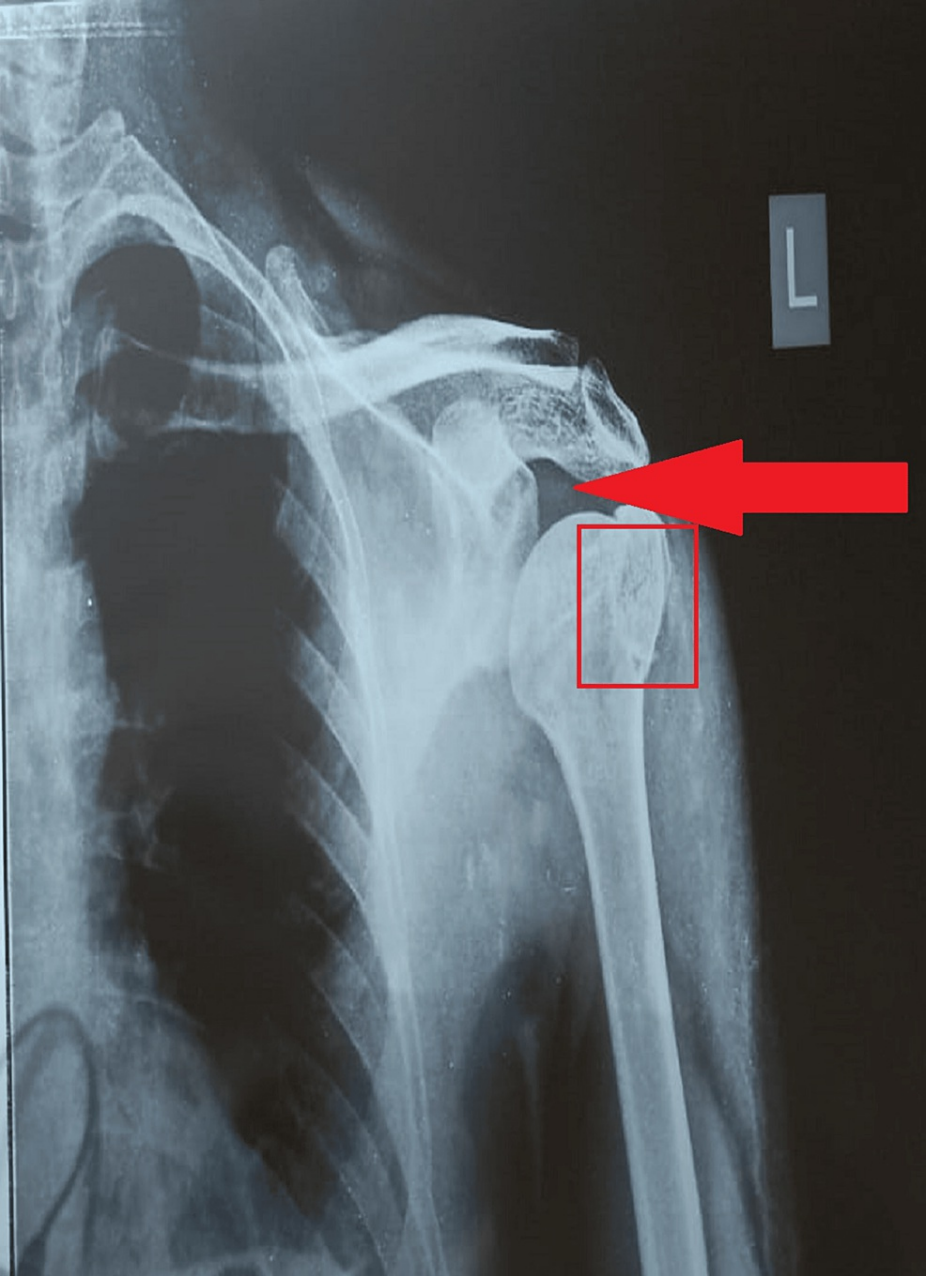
Preoperative anteroposterior X-ray of the left shoulder joint presenting a fracture of greater tuberosity of the humerus with slight displacement. The arrow depicts the displacement of the humeral head and the box depicts the fracture of greater tuberosity.
